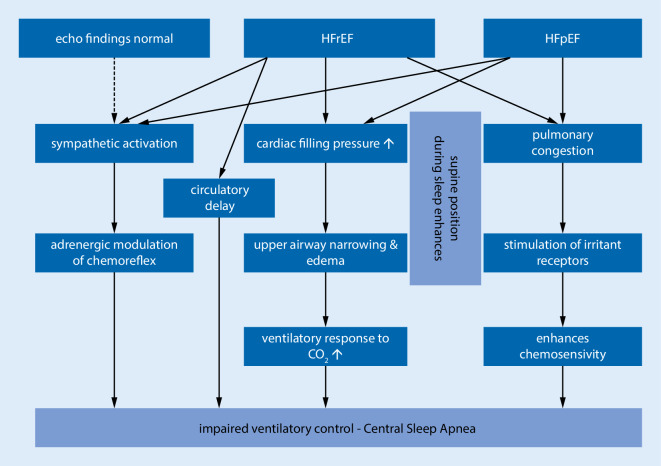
Direct mechanical effects and loss of neuromuscular control mechanisms together contribute to the pathophysiology of central sleep apnoea (CSA). Several risk factors associated with CSA are not explained by these pathways (e.g., male gender, age). Sympathetic activation seems to be the only mechanism that may contribute to CSA in patients with normal echocardiographic findings. It is also the only mechanism that is shared by the three different heart conditions. *HFrEF* heart failure with reduced ejection fraction, *HFpEF* heart failure with preserved ejection fraction
